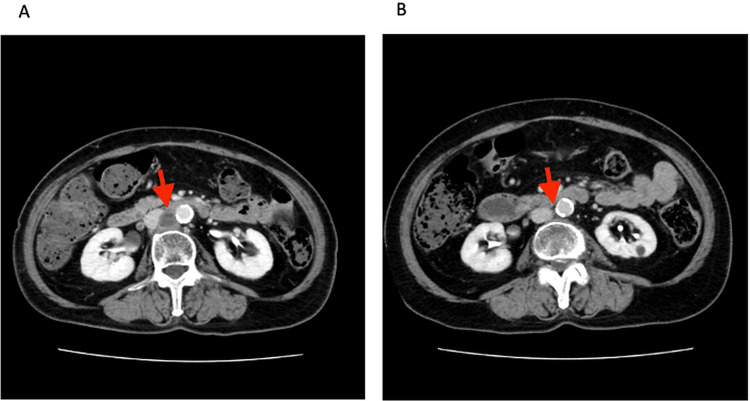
Computed tomography (CT) findings for the response to pembrolizumab treatment. (A) CT demonstrating multiple enlarged lymph nodes (red arrow) in the para-aortic regions after adjuvant chemotherapy. (B) Follow-up CT showing complete radiologic response after pembrolizumab therapy (red arrow).
